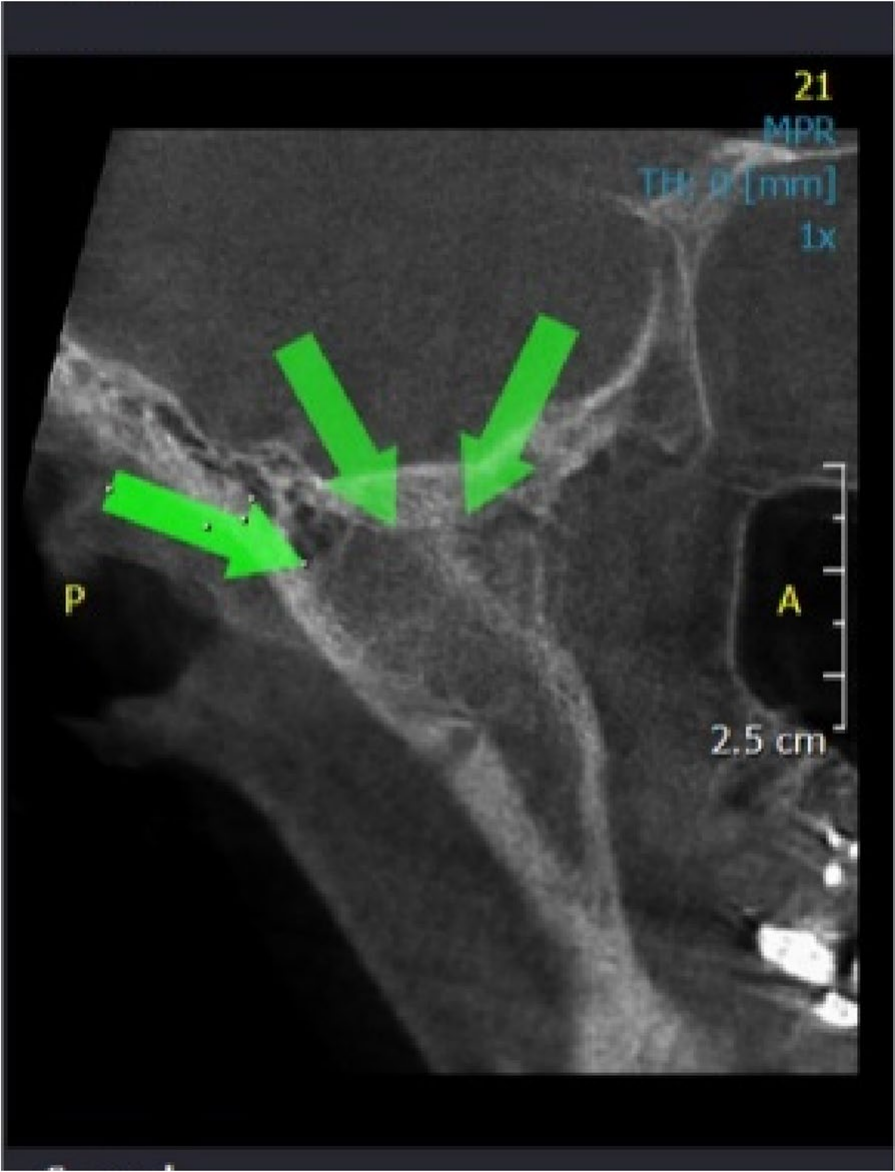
Coronal CBCT view showing condylar Ankylosis
